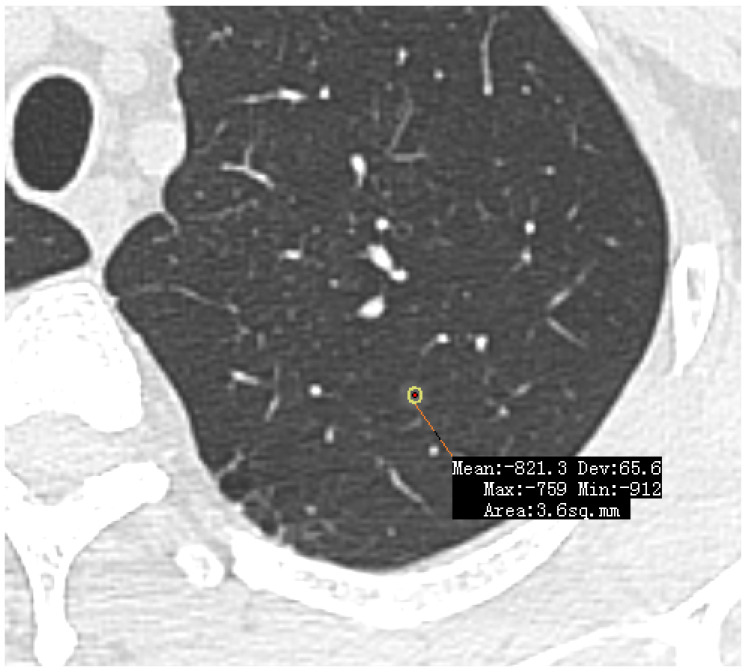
Example of an ultra-low density false negative nodule. An axial HRCT view depicting a faint GGO in the apicoposterior segment of the left upper lobe, missed by both CAD systems. Due to its extremely low CT attenuation (measured at −821 HU), the grayscale difference between the nodule and the surrounding normal lung parenchyma is minimal, making detection highly challenging for standard algorithms. The yellow circle with a red dot represents the manually placed region of interest (ROI) used to measure the mean CT attenuation (−821.3 HU).
